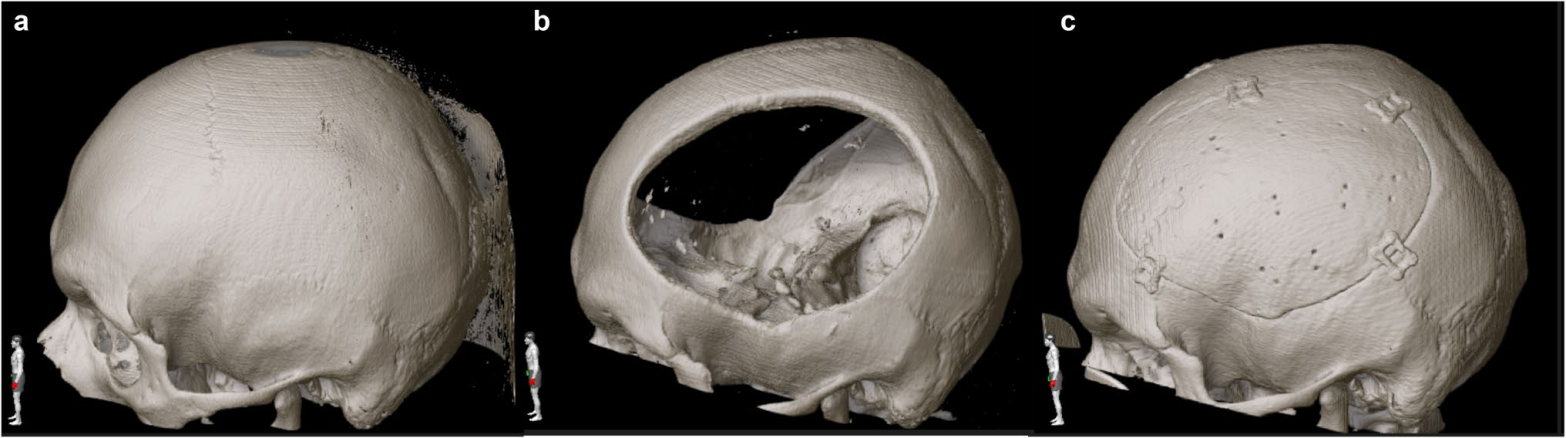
3D reconstruction of cranial CT scans before craniectomy (a), after craniectomy (b) and after cranioplasty (c) in a patient with bilateral decompressive craniectomy
